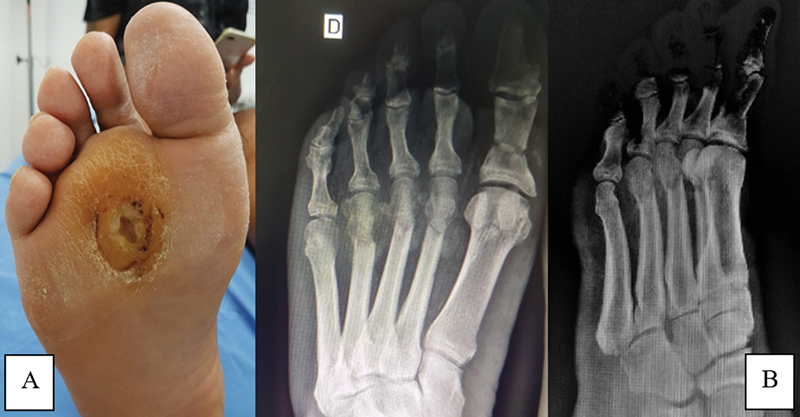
Clinical and radiographic appearance of the right foot in the third postoperative week. (
